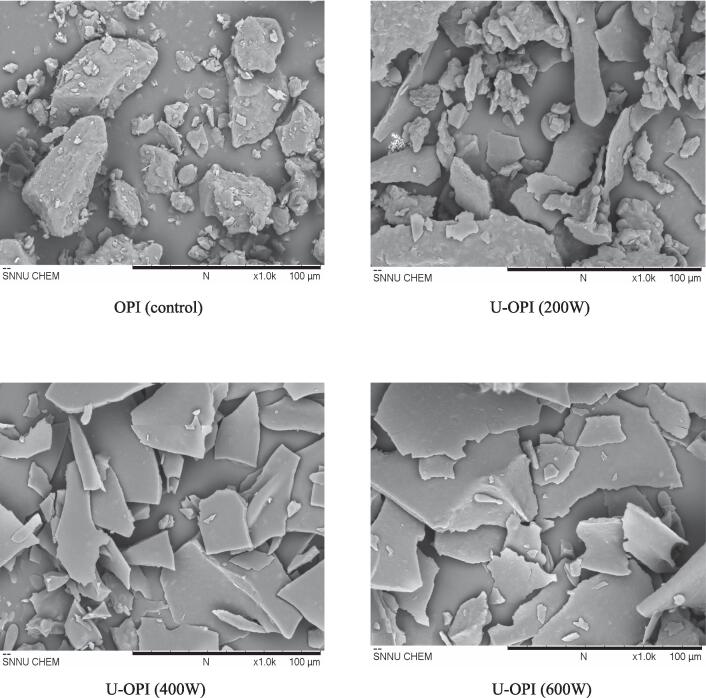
Impact of ultrasound-treatment on micro-structure of oat protein isolates (SEM).
